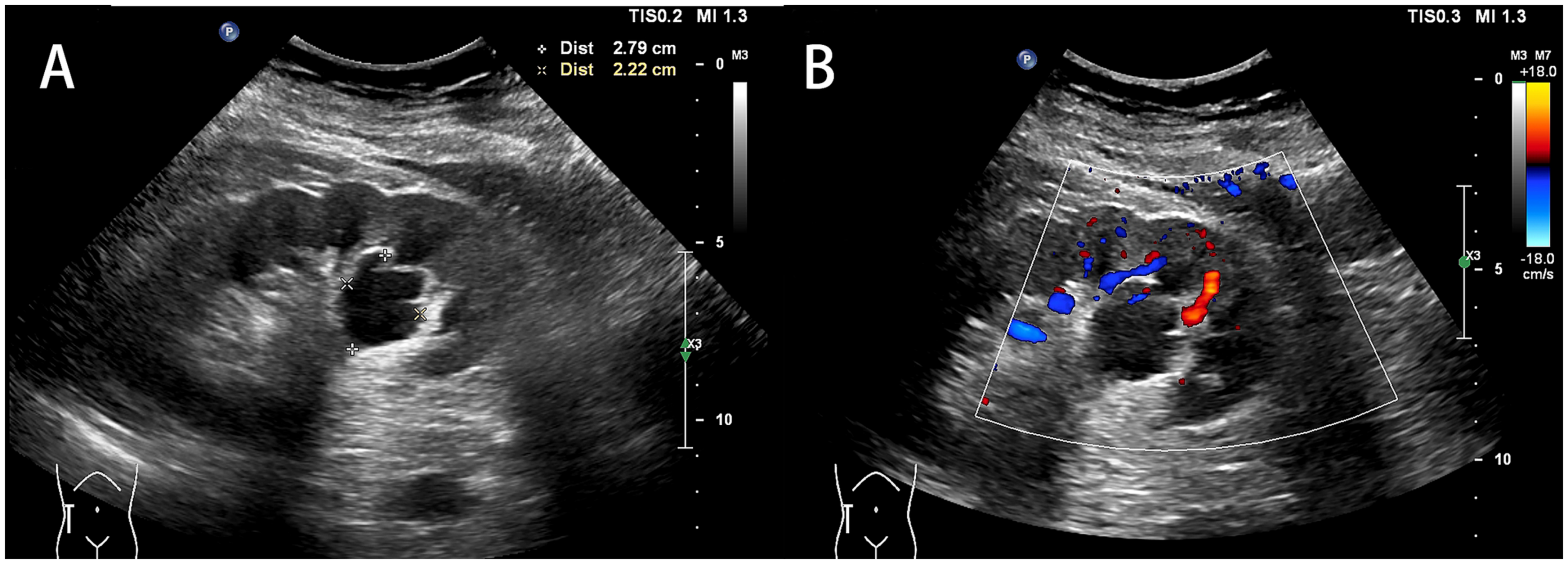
Ultrasonographic features of IgG4-related kidney disease mimicking renal cell carcinoma. (A) Grayscale (2D) ultrasonography reveals a relatively well-defined, heterogeneous, hypoechoic mass-like lesion in the kidney, distorting the normal renal architecture. (B) Color Doppler flow imaging shows some peripheral blood flow around the mass, but no definite vascular signals are detected within the lesion itself.
